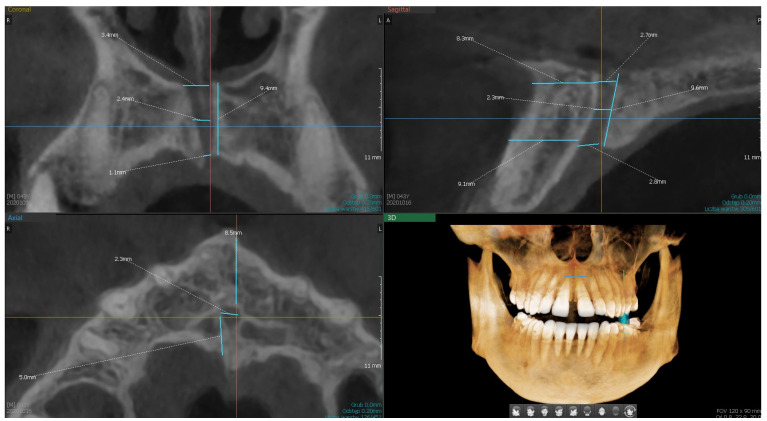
CBCT images for assessment and measurements of the regional morphology, available bone, and accessory canals in three radiological sections (axial, sagittal, coronal).
